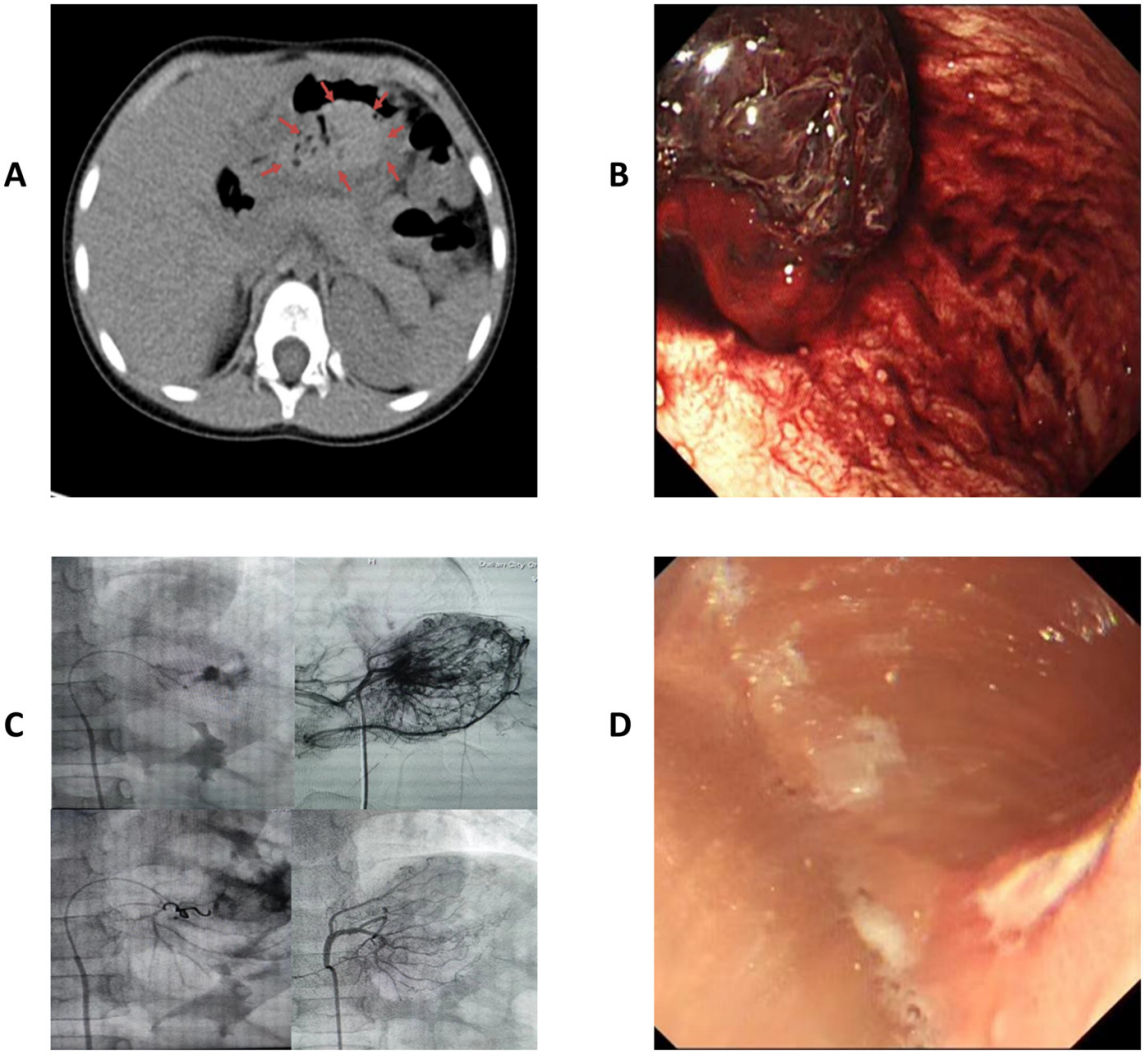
(A) Outpatient computed tomography showing a gastric space-occupying lesion. Arrows circumscribe the hematoma. (B) Gastroscopy revealed a gastric hematoma with progressive bleeding. (C) Interventional embolization for hemostasis. (D) Multiple gastric ulcers in follow-up gastroscopy 8 d postoperatively.
